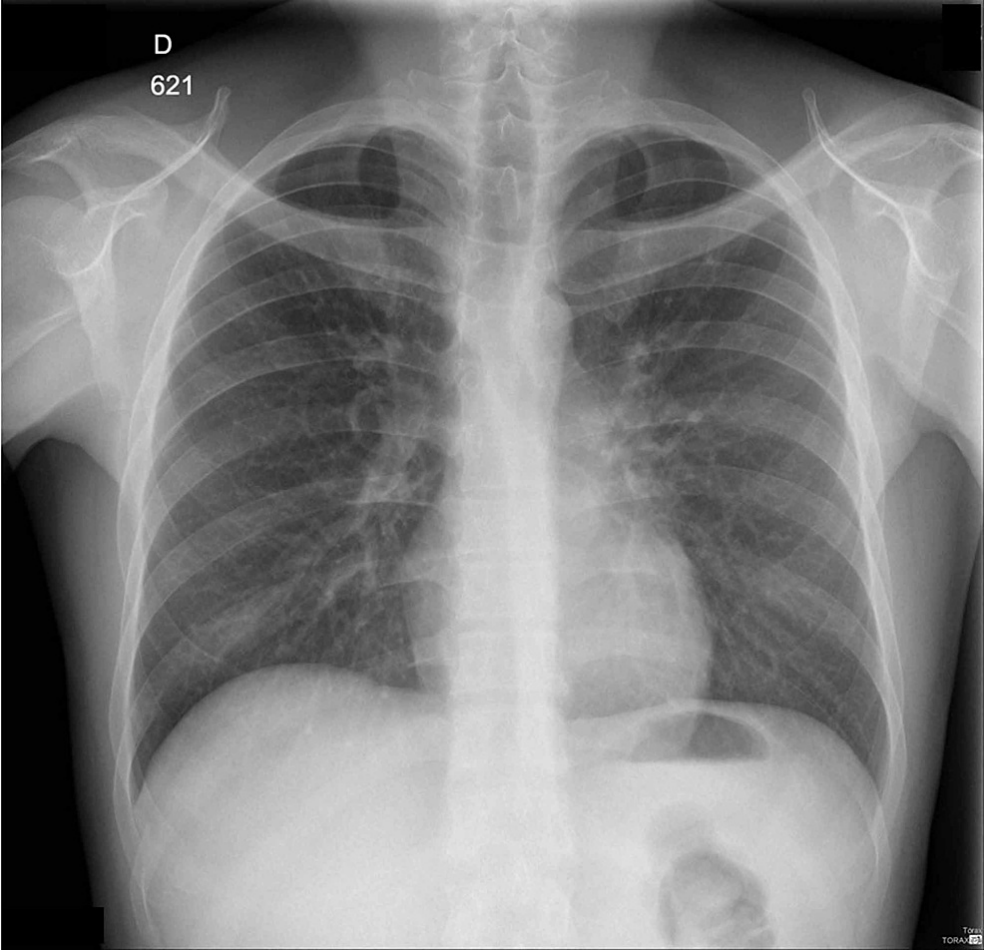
Chest X-ray on admission Chest x-ray on admission showing no infiltrates suggestive of pulmonary disease.
